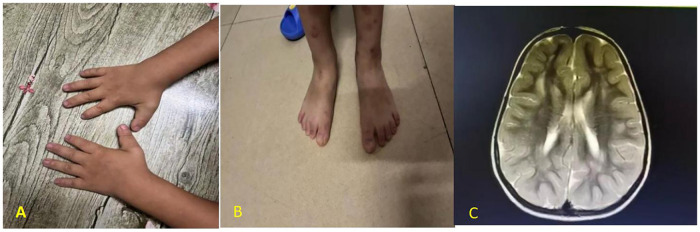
(A) characteristics of our pediatric patient's hands and feet (at 4 years of age); (B) note his small hands and feet, accompanied by brachydactyly. (C) Cranial MRI findings: Bilateral periventricular white matter lesions with dysmyelination.
